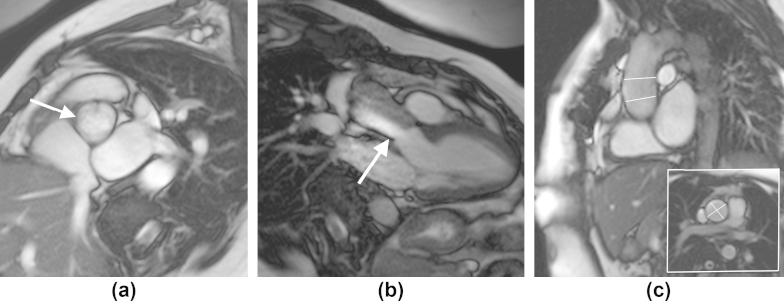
Systolic bSSFP cine MRI of the aortic valve (a), the aortic root (b) and ascending aorta (c,d) in a 45-year-old TS patient with mild aortic stenosis and aortic regurgitation on transthoracic echocardiography, which was unable to assess the aortic dimensions. (a) Atrioventricular view demonstrates a L-R BAV (arrow), and (b) the three-chamber view shows an asymmetric jet flow through the BAV (arrow). (c) Shows the aortic root and ascending aorta in sagittal oblique (diameter measurements white lines) and left bottom figure (white frame) in axial views at the level of the main pulmonary artery.
